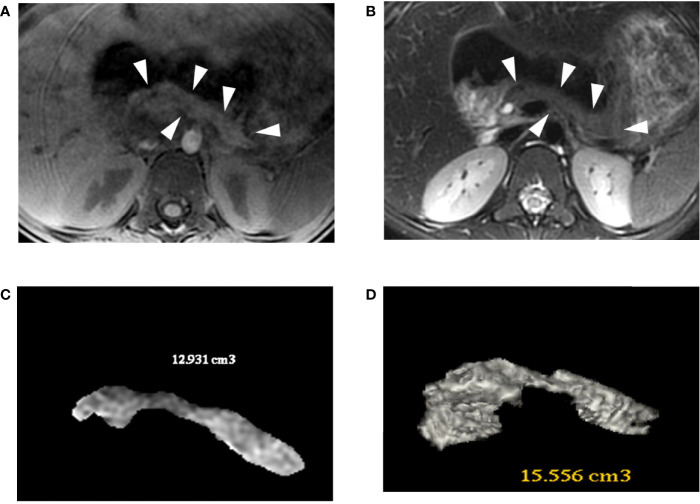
Patient 2 (R181W) 3D-MRJ T2 (A) and Tl-weighted (B) with volume rendering reconstructions (C, D). The MRI shows a small pancreas (white arrowheads), especially in the bod y and tail, with a volume estimated between 13 and 15.5 cc. For comparison, an aged-matched control patient has a pancreatic volume of 45 cc.
